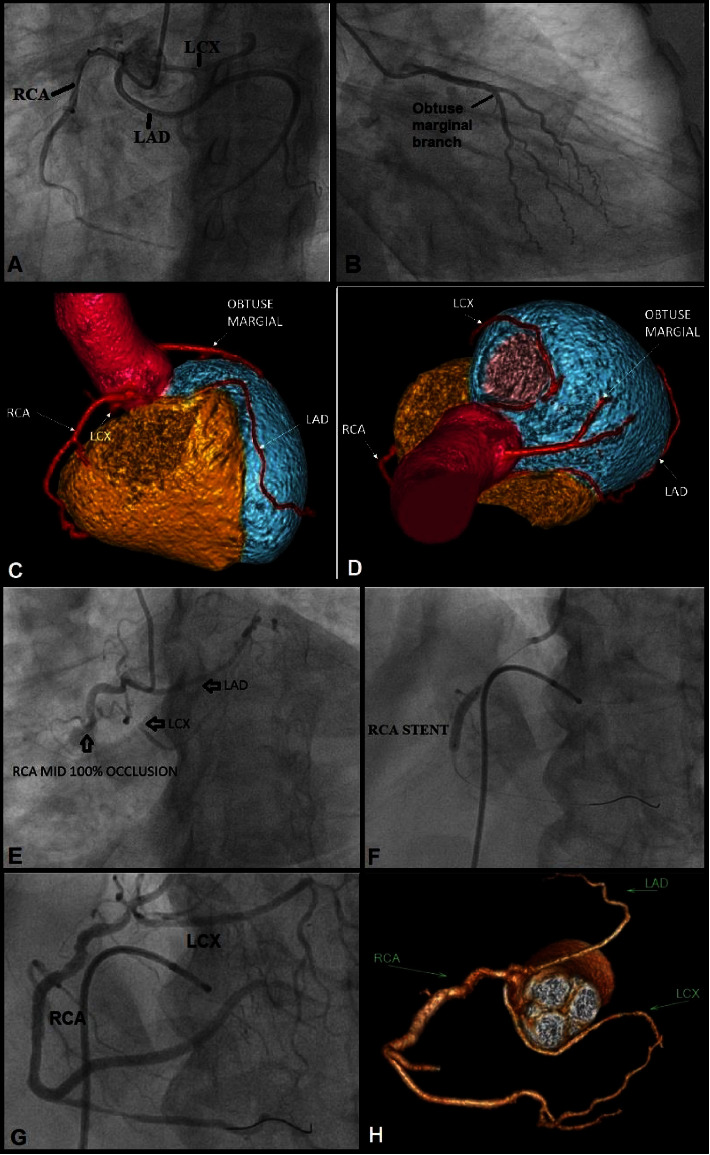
(a): Coronary angiogram showing the origin of all 3 major coronaries from the right coronary sinus via common ostium. (Case 1). (b): CAG showing a branch arising from the left sinus supplying obtuse marginal territory. (Case 1). (c) & (d): 3D volume-rendered reconstructed CT image showing the origin of LAD, LCX, and RCA from the right sinus of Valsalva via common ostium and origin of the small obtuse marginal branch from the left sinus of Valsalva. (Case 1). (e): CAG showing the origin of all 3 major coronaries from the right sinus along with complete occlusion of RCA and 90% occlusion of LCX. (Case 2). (f): Image showing the stent in RCA along with temporary pacemaker lead in the right ventricle. (Case 2). (g): Post angioplasty image showing TIMI-III flow in RCA and LCX. (Case 2). (h): Volume-rendered coronary tree showing the common origin of all 3 major coronaries from the right sinus. (Case 2).
